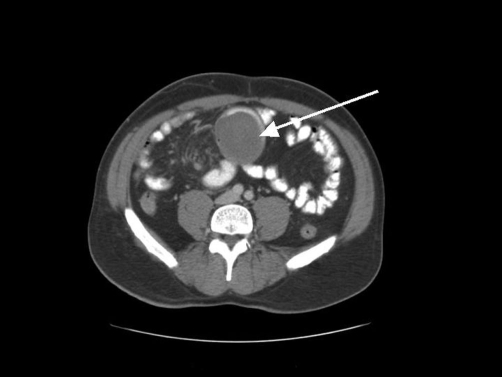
Enhanced CT scan of abdomen and pelvis demonstrating a well defined soft-tissue mass arising from the jejunum.
